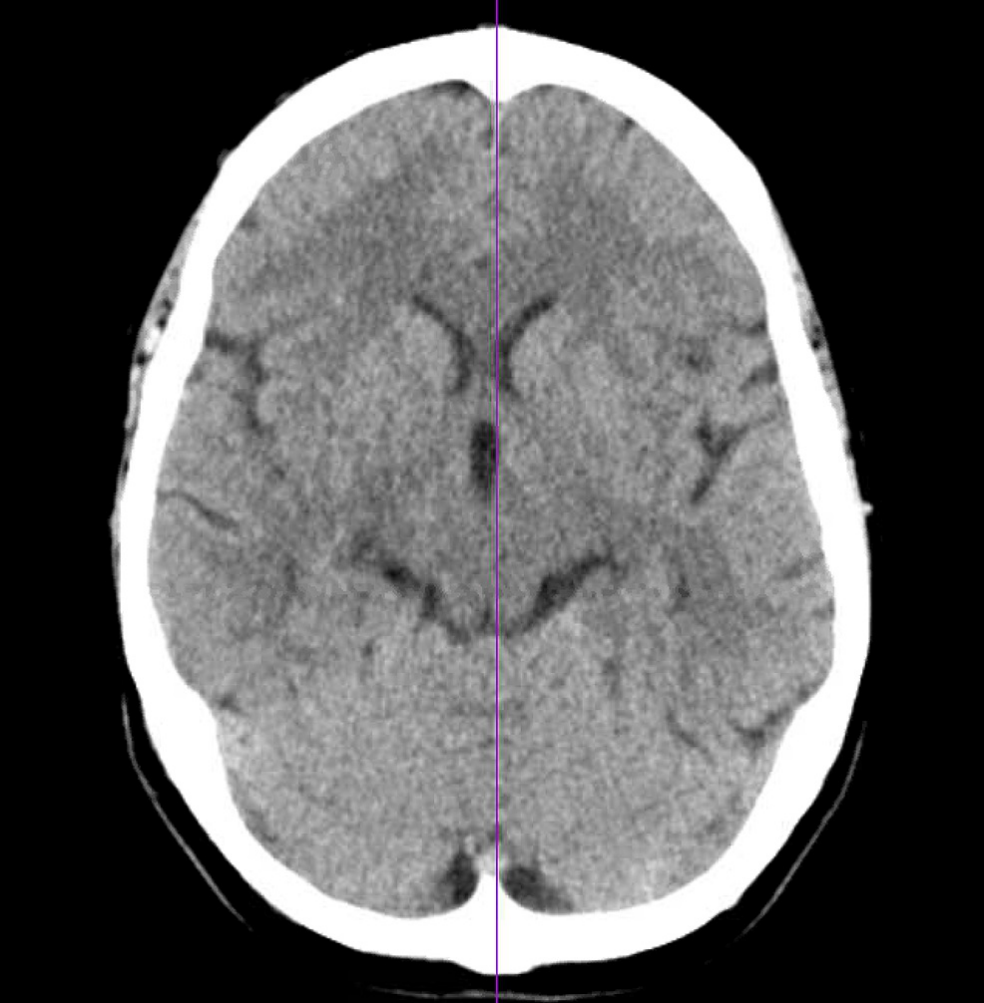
Computerized tomography scan of the brain. The computerized tomography (CT) scan of the brain showed no acute intracranial bleed, midline shift, or mass effect.
